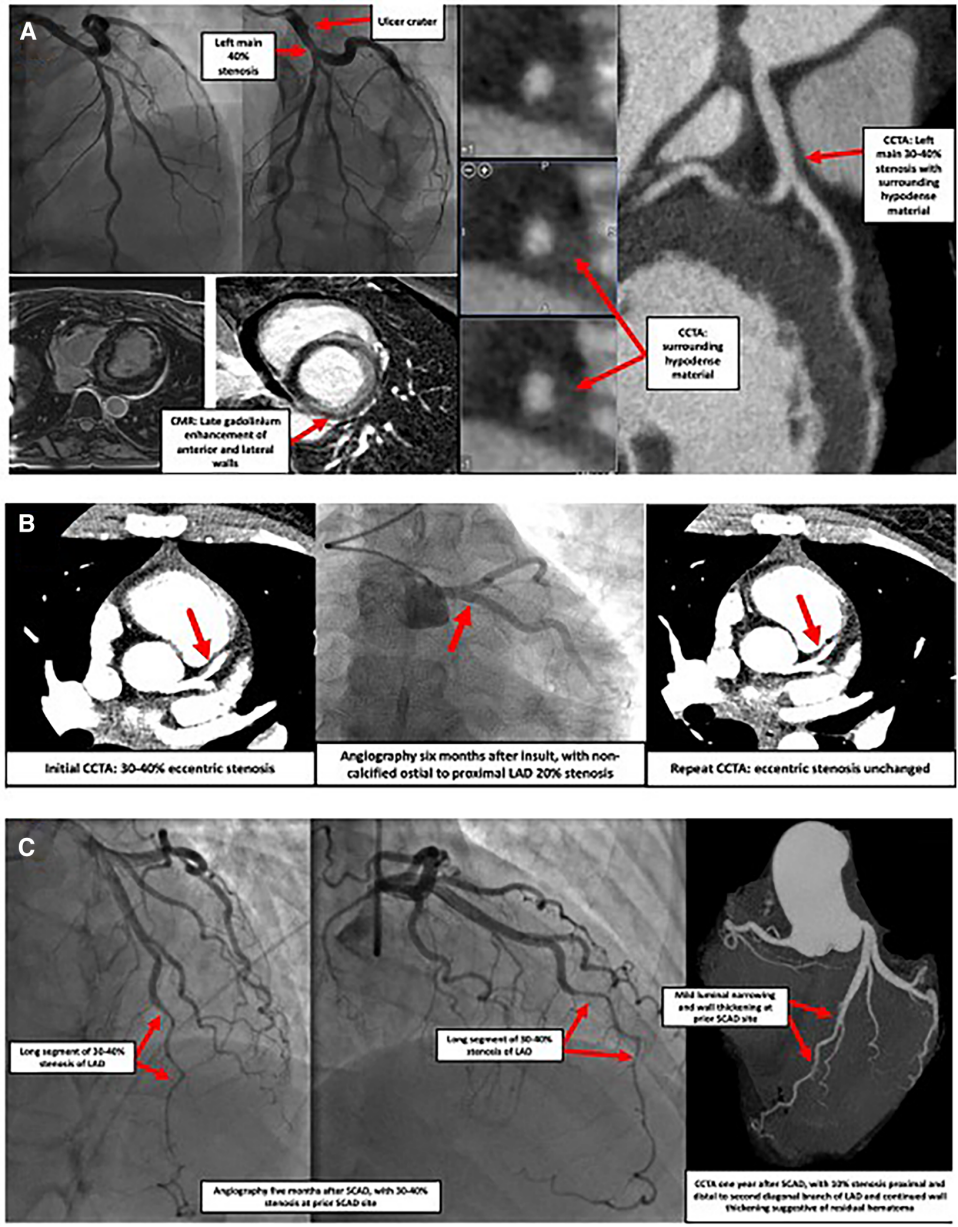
Images of three separate cases showcasing SCAD presentations. (A) Case of a 42-year-old female who presented with syncope, ventricular tachycardia, and elevated troponin. Coronary angiography indicated an ulcer crater in the left main coronary artery with 40% stenosis which extended into the origin of the LAD and the LCx (top left panel). Cardiac MRI showed delayed transmural gadolinium enhancement in the anterior and lateral walls as well as the lateral aspect of the inferior wall, suggestive of a vascular insult (bottom left panel). Subsequent coronary CTA found 30%–40% stenosis of the distal left main, with surrounding hypodense material causing vessel enlargement, suggestive of SCAD with intramural hematoma (right panel). Similar findings were seen in the very proximal portions of the LAD and LCx. No atherosclerosis was observed. LAD, left anterior descending coronary artery; LCx, left circumflex coronary artery; MRI, magnetic resonance imaging; CTA, computed tomography angiography; SCAD, spontaneous coronary artery dissection. (B) Case of a 30-year-old female with fibromuscular dysplasia who presented with chest pain. CCTA (left panel) showed a 30%–40% stenosis of the ostial to proximal LAD, consistent with a coronary dissection. Coronary angiography (middle panel) and repeat CCTA (right panel) performed six months later showed a focal eccentric 20% ostial LAD stenosis compatible with healed SCAD or intramural hematoma. CCTA, coronary computed tomography angiography; LAD, left anterior descending coronary artery; SCAD, spontaneous coronary artery dissection. (C) Case of a 53-year-old female who presented with an acute anterior MI and was found to have SCAD of the mid-to-distal LAD (left panel). Five months later, recurrent chest pain prompted repeat coronary angiography, discovering a focal myocardial bridge and a long region of 30%–40% stenosis at the prior SCAD site (middle panel). No interventions were undertaken. One year after the initial event, another episode of chest pain prompted CCTA, which showed a larger lumen, with only mild 10% stenosis at the prior SCAD site with continued vessel wall thickening suggestive of residual hematoma (right panel). SCAD, spontaneous coronary artery dissection; LAD, left anterior descending coronary artery; CCTA, coronary computed tomography angiography).
